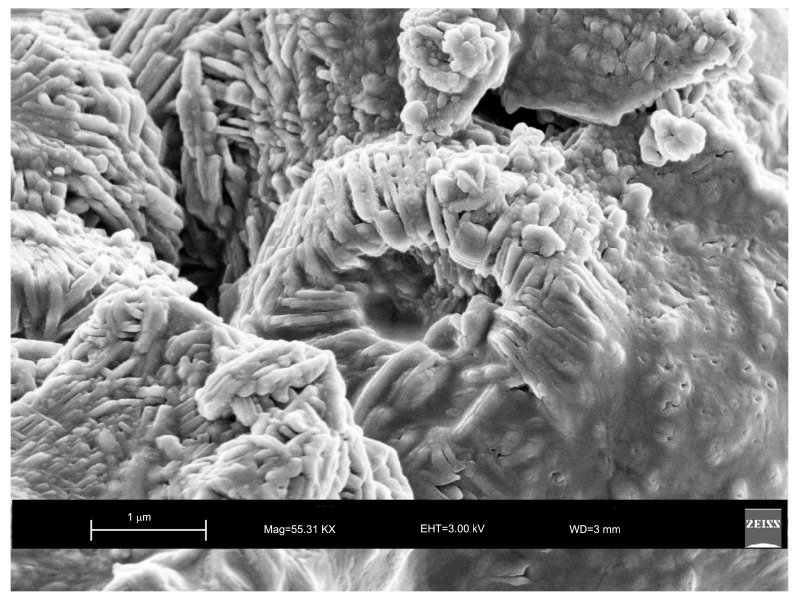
SEM image showing well defined plate morphology of sodalite crystal formed 6 months after hydrothermal treatment with ultrasonic.
